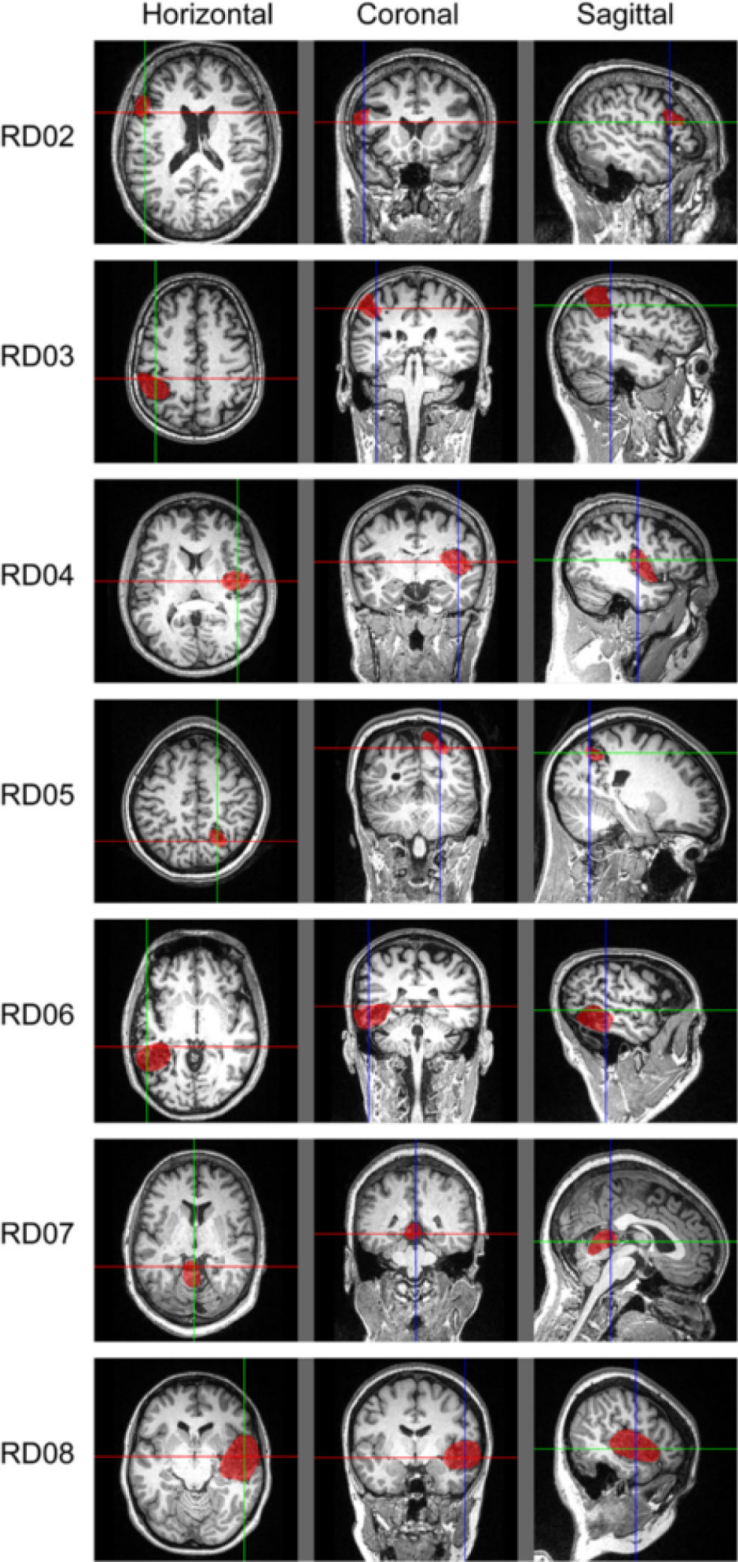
AVM Location on T1-weighted Horizontal, Coronal and Sagittal MRIs in anatomical space. Image right is anatomical right. For each subject, the pre-surgical T1w MRIs are displayed. Colored lines orient location of each MRI view: green runs front-back, red runs left-right and blue runs up-down. AVMs were traced for distance-from-AVM analysis and the inside of the AVM is shaded red for visualization
